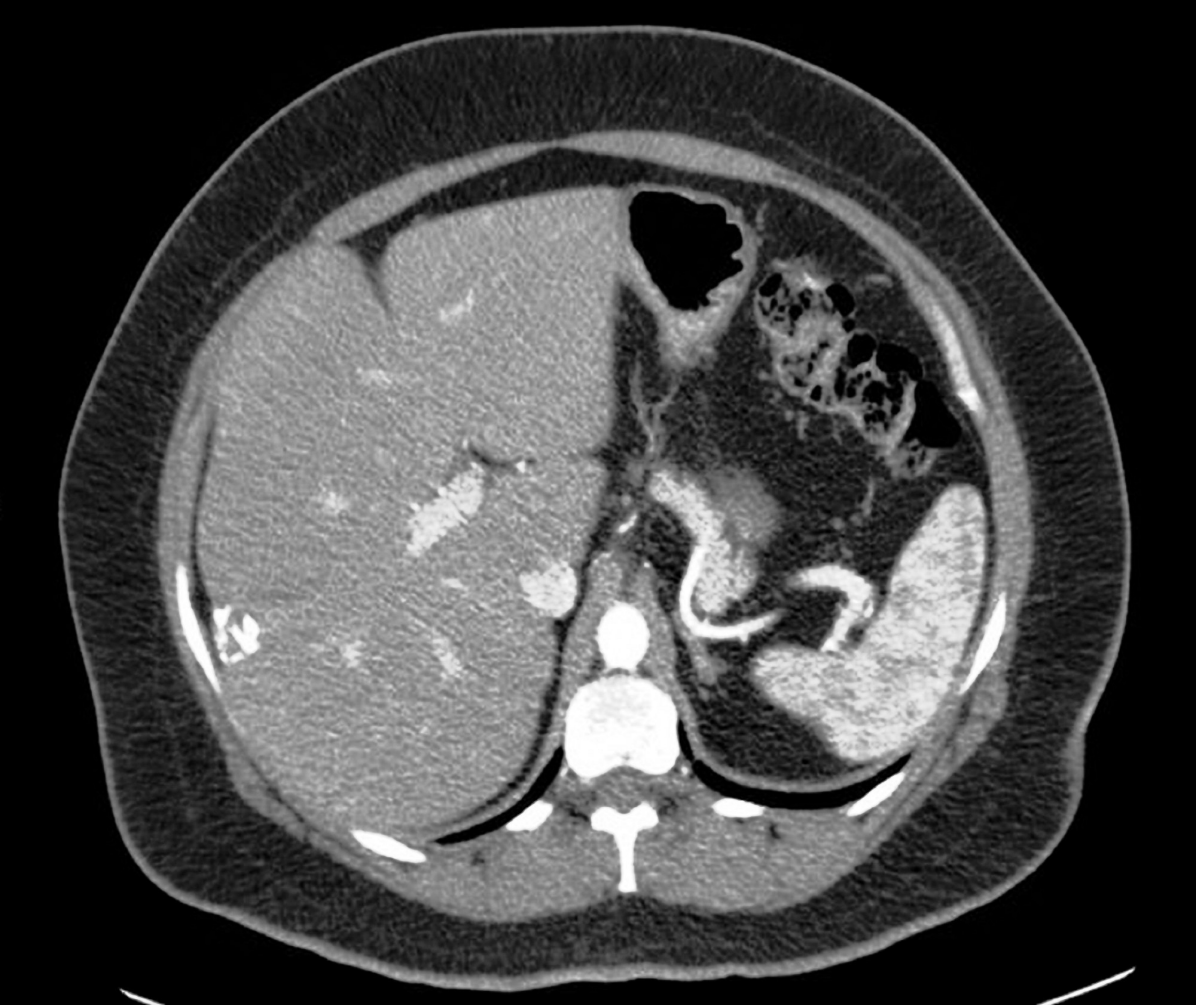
CT on follow-up after one year The previously treated lesion in segment VI did not reveal a significant change in size and had no enhancement.
